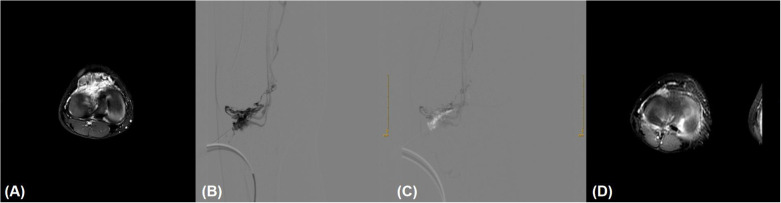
A 9-year-old girl with venous malformation of the right knee who underwent sclerotherapy under DSA guidance. (A) Axial fat-suppressed T2-weighted magnetic resonance imaging of the right knee reveals hyperintense venous malformation lesion. (B) DSA angiography after local puncture of the lesion revealed the malformed venous mass and draining veins. (C) Injection of polidocanol sclerosant. (D) Postoperative follow-up MRI showed significant regression of the lesion.
